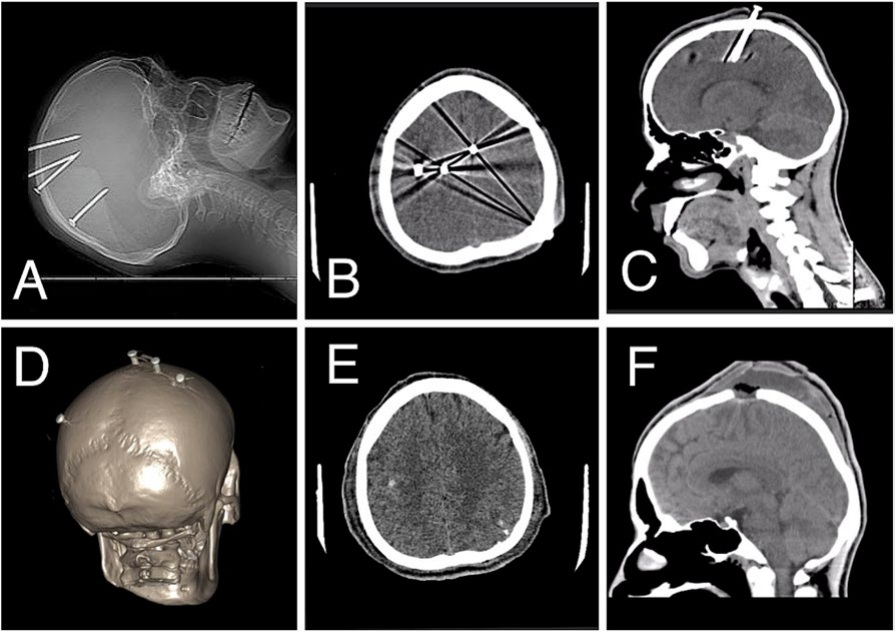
A, B Pre-operated CT brain plain axial view showing penetrating objects. C Pre-operative sagittal CT brain plain showing ¼ foreign penetrating object not associated with underlying hemorrhage. D Three-dimensional Computed tomography skull showing all 4 penetrating objects, 1 penetrating left parietal bone, and the remaining 3 penetrating right parietal bone. E Post-operative axial CT brain plain, where Post-operative pinpoint hemorrhage can be seen. F Post-operative CT brain plain sagittal view showing craniectomy done around penetrating object and subgaleal collection. No Postoperative intraparenchymal hemorrhage was seen
